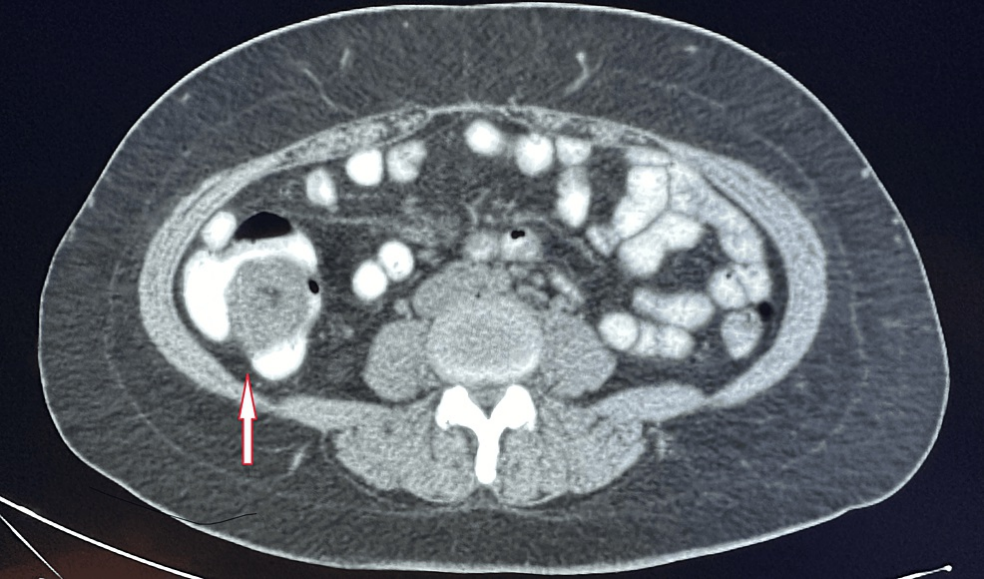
CT scan of the abdomen An arrow pointing to the cecal intussusception.
